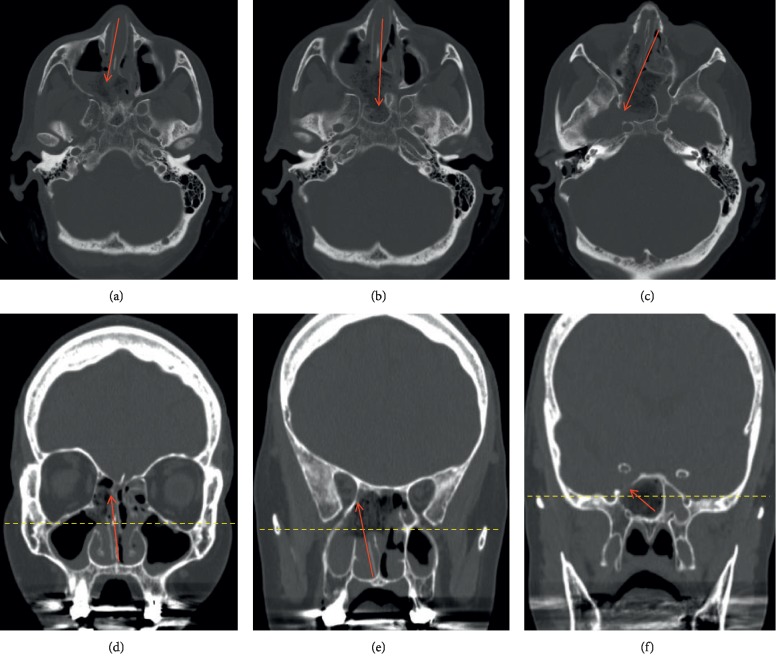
CT maxillofacial with sequential cuts to demonstrate the overall trajectory of an endoscopic endonasal right transpterygoid approach. (a–c) Axial views of the anterior skull base. (d–f) Coronal views of the same region. Red arrows estimate the working trajectory. Note the bony opening in the anteromedial aspect of the middle cranial fossa to expose the contents of MC in axial (c) and coronal views (f). This corresponds to the intraoperative view in Figure 4 with the internal carotid artery located superior and medial to the bony opening.
